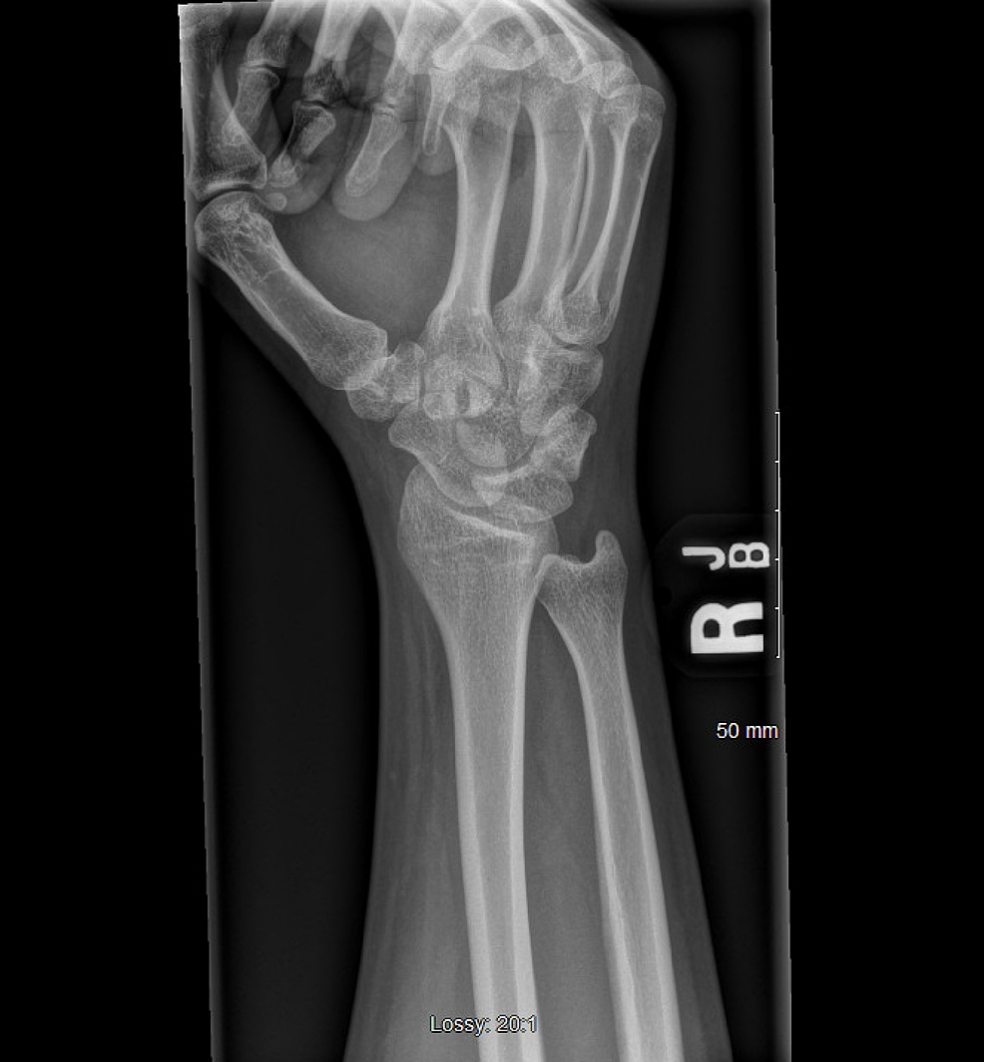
Oblique X-ray of the patient's right wrist demonstrating normal findings
